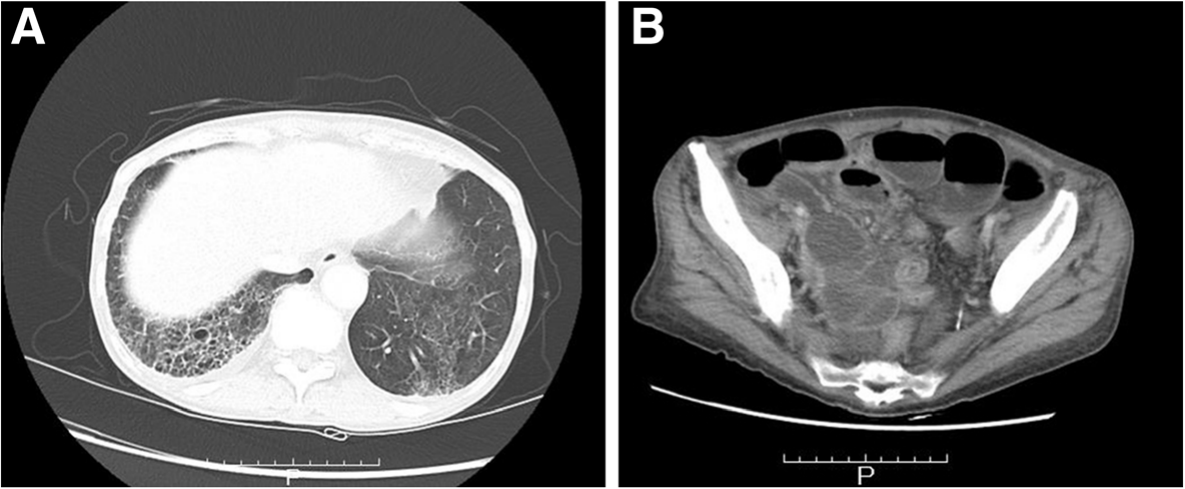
Computed tomography (CT) findings. A: Chest CT scan showed honeycomb changes and ground-glass opacities in the lung. These changes suggested interstitial pneumonia. B: Abdominal CT scan showed dilatation and wall thickening of the small intestine.
